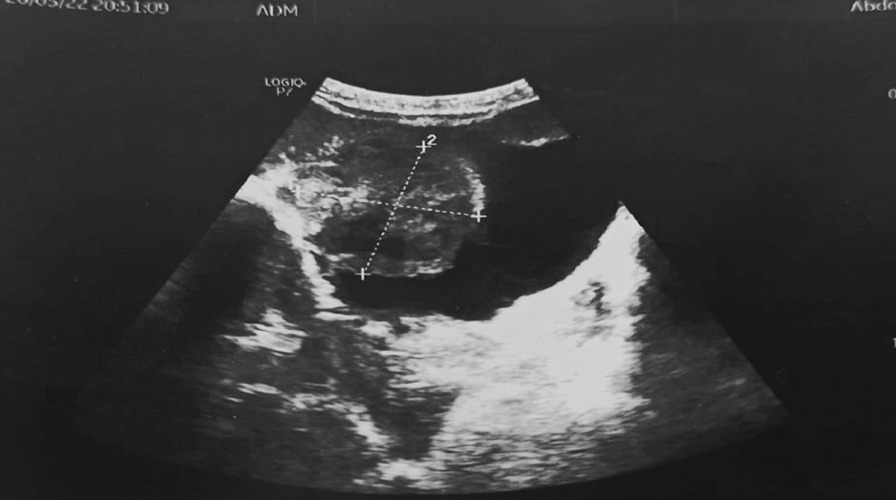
Gray-scale ultrasound image of the urinary bladder shows a calcified heterogeneous 62 × 54 mm mass along the right lateral wall of the bladder
